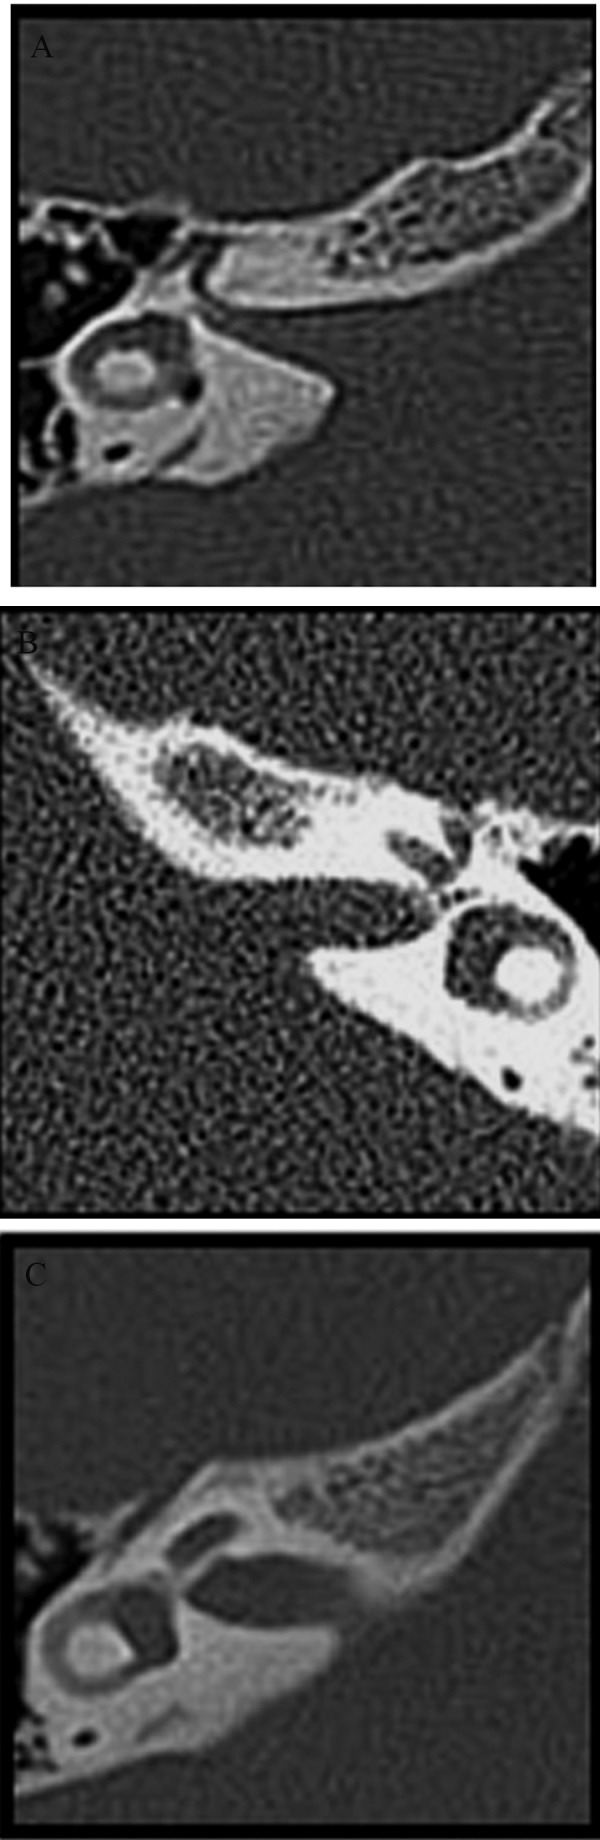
Types of shapes observed for the IAC in CT images A) Funnel-shaped, B) Cylinder-shaped, C) Bud-shaped
